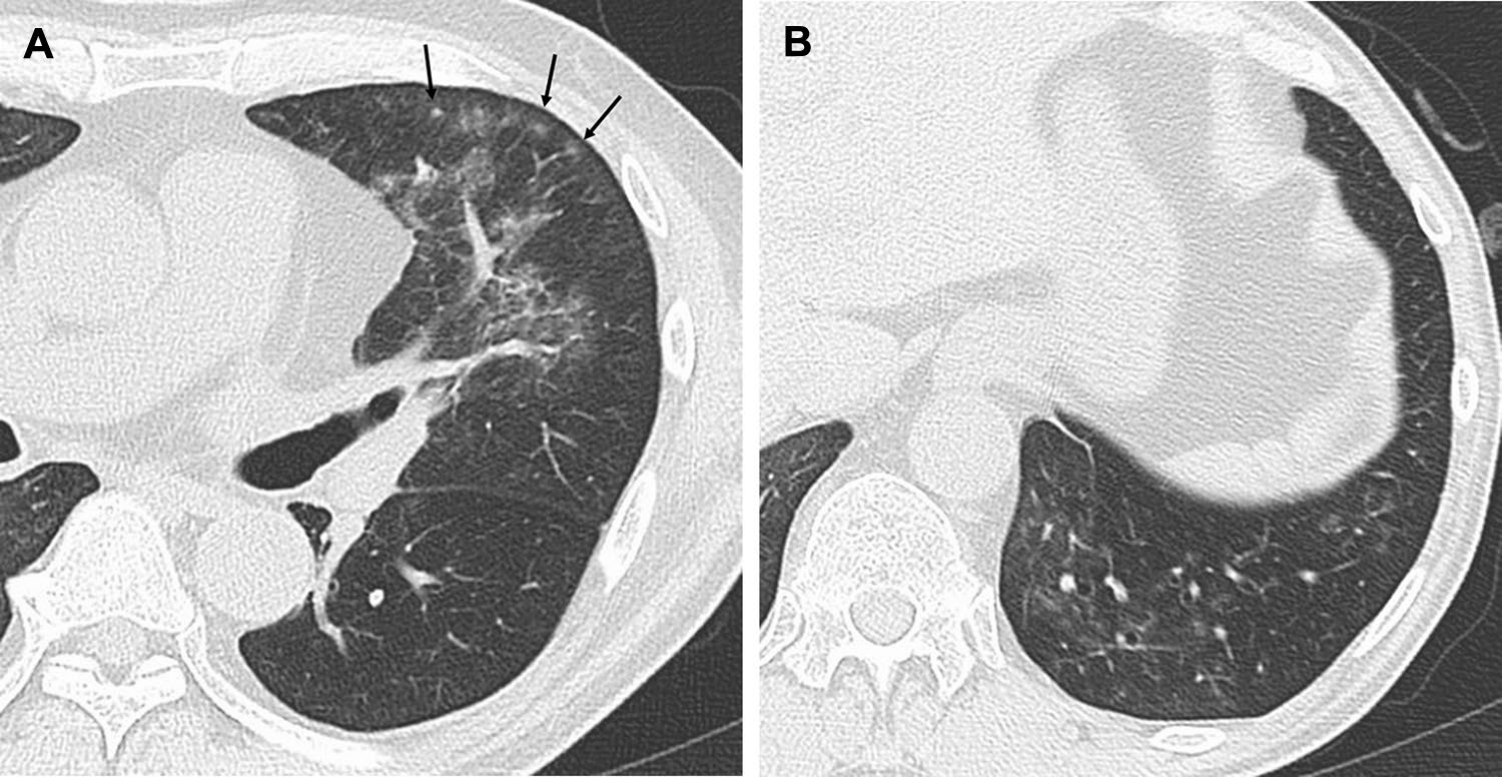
A 59-year-old woman with pneumocystis pneumonia under treatment for malignant lymphoma after hematopoietic stem cell transplantation. A High-resolution CT shows ground-glass attenuation with small nodules with centrilobular distribution (arrows). B High-resolution CT shows small ground-glass nodules
